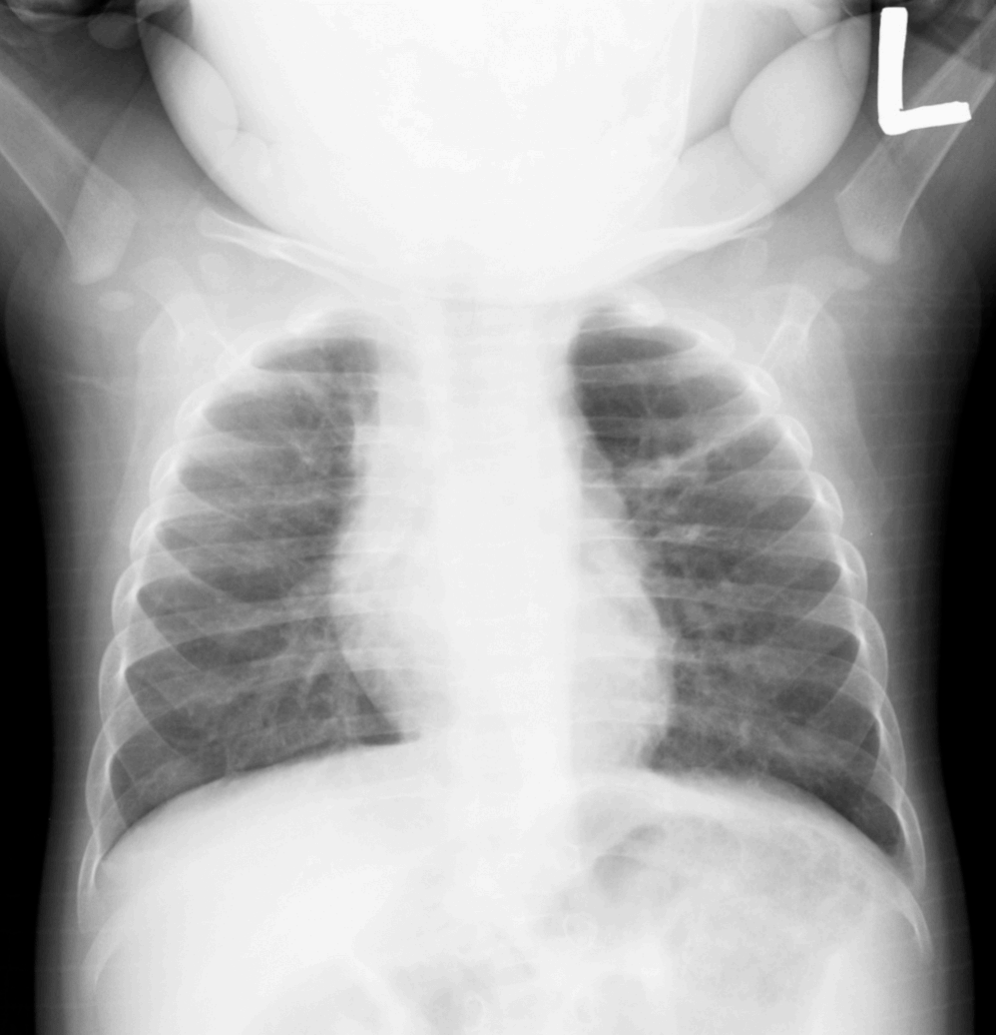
Chest radiograph at the age of 1 year showing similar increased radiolucency in the left upper lung field.
